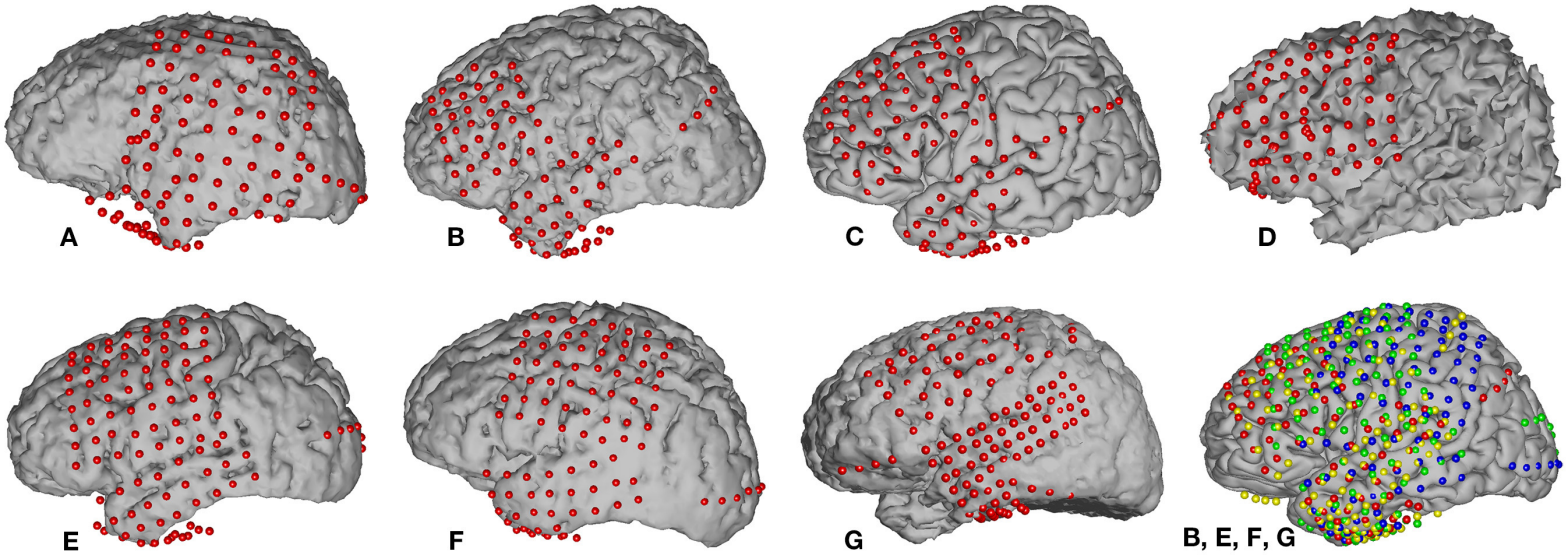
Locations of implanted grids on individual subject cortical models based on co-registered pre-op MR and post-op CT data. The bottom right figure shows the electrode locations projected on an average brain for those four subjects (B, E, F, and G) that passed initial screening (subjects A, C, and D were not included, see Section 2.3.5). Each subject's electrodes are represented with a different color.
